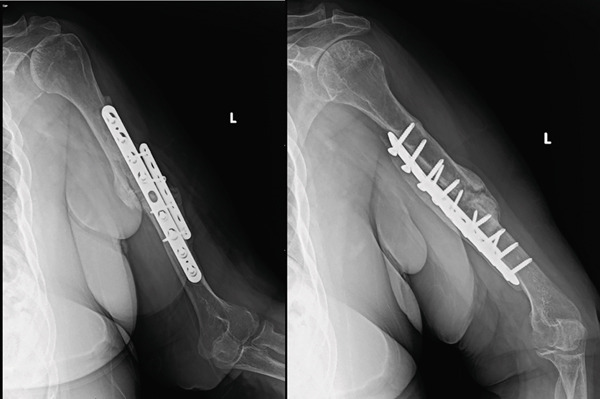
Radiographs taken 5 months postoperatively, 8 months after the initial injury demonstrating a healed humerus fracture with acceptable alignment.
